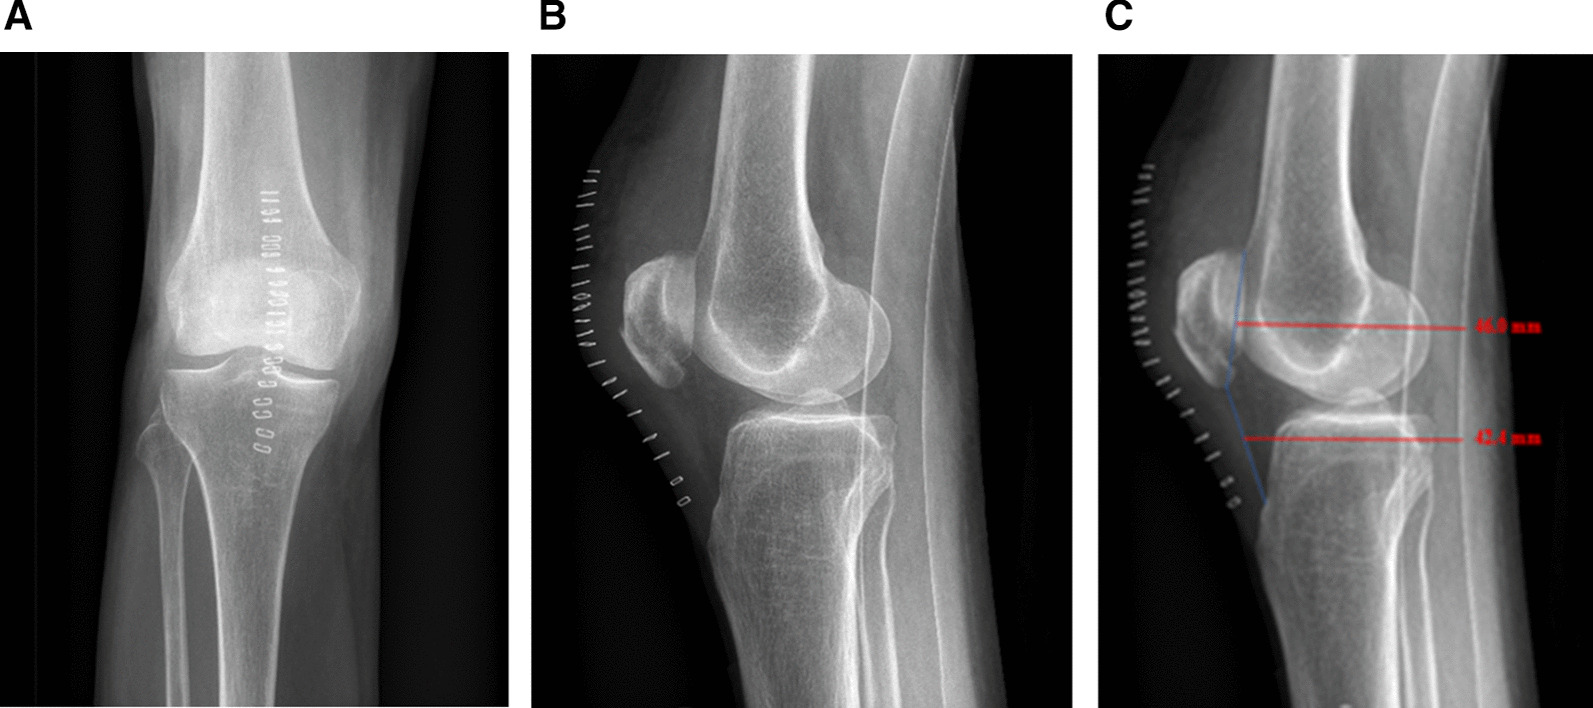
a, b, c: Day one post operative control x-ray—Insall-Salvati ratio = 0,92
